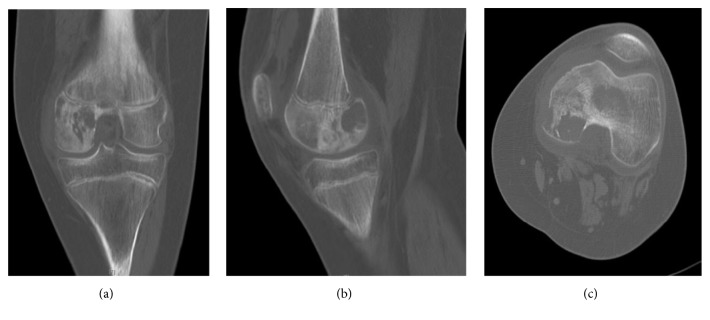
Computed tomography (CT): coronal (a), sagittal (b), and axial views (c) of the left knee, July 2009.
